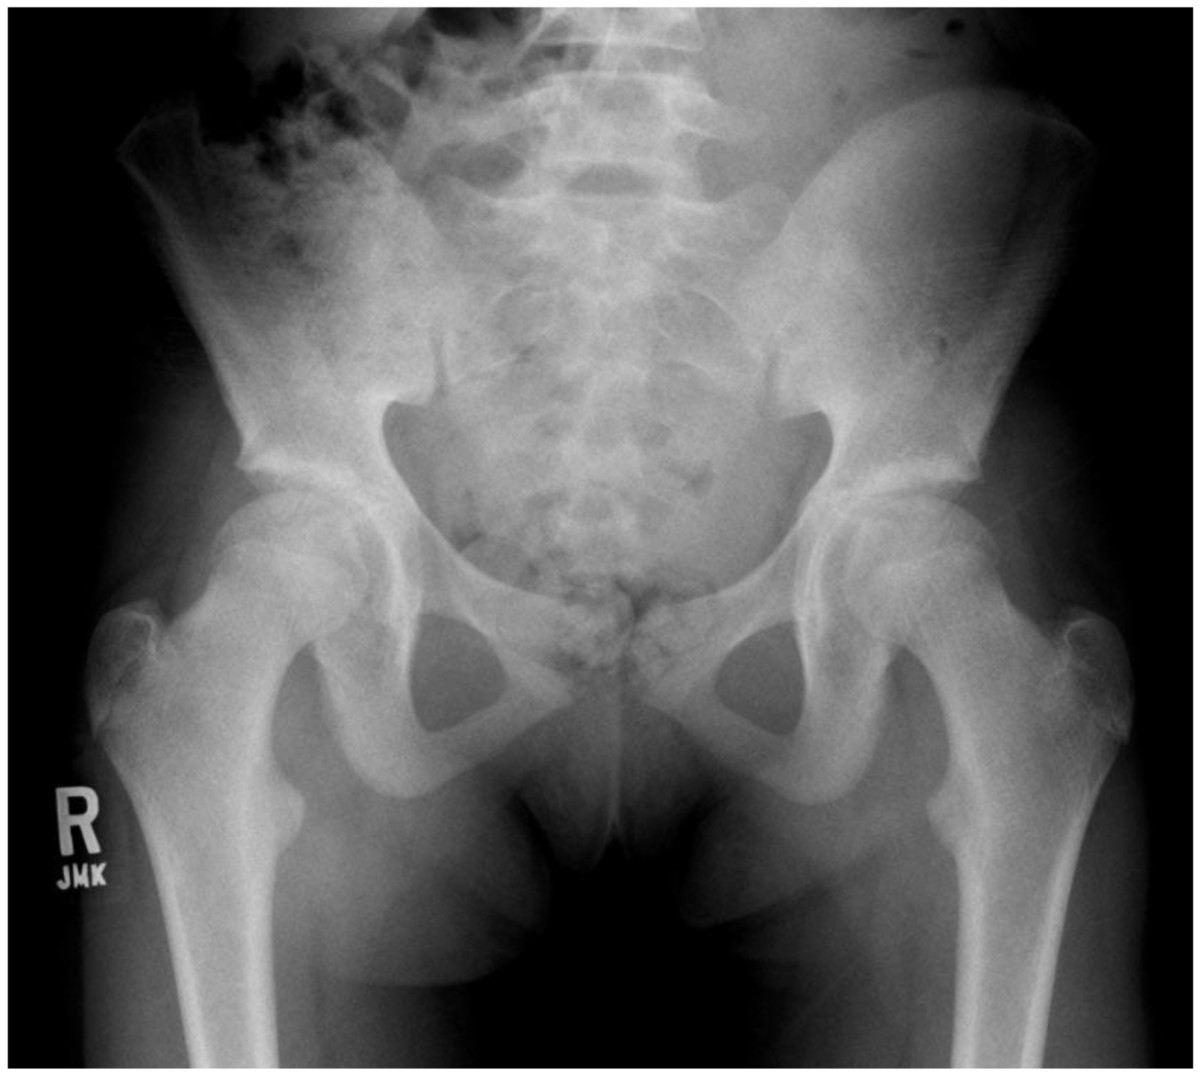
Anteroposterior radiograph of the pelvis of the additional insightful patient at age 12 years. The outward margin of the right acetabulum is tilted upward, and no secondary centers of ossification are present in the hip.
